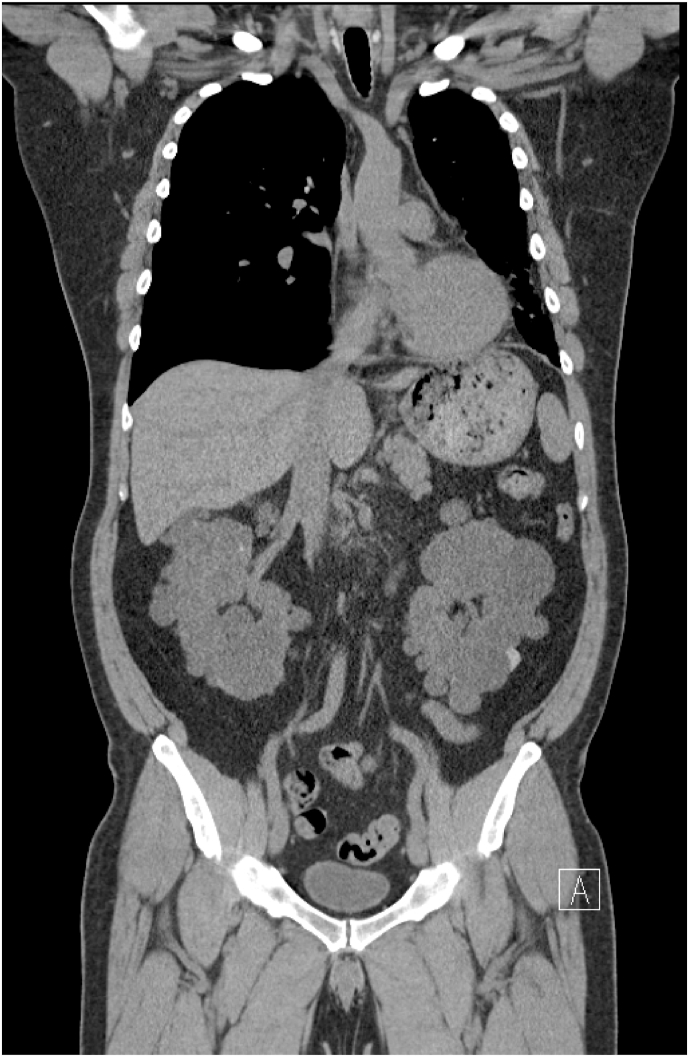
CT scan of the abdomen bilateral extensive kidney cystic disease consistent with patient's history of ADPKD.
